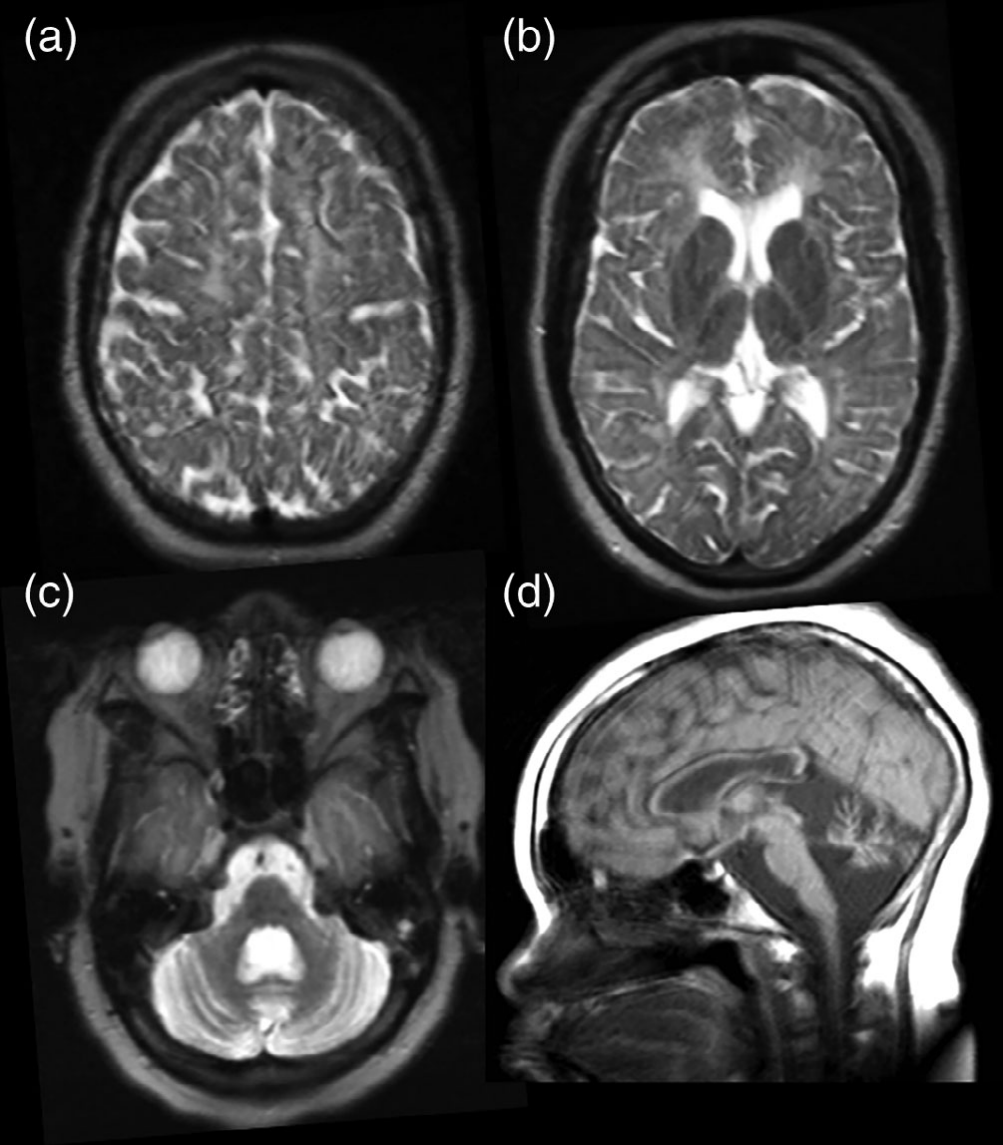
This brain MRI was performed at the age of 17 years. (a–c) are T2‐weighted axial images, demonstrating diffuse hyperintense signal of the white matter, mild supratentorial atrophy, and severe cerebellar atrophy. The ventrolateral thalamus (b) and the medial lemniscus (c) are relatively hypointense, as often seen in 4H leukodystrophy. The sagittal T1‐weighted image (d) demonstrates cerebellar atrophy and a thin corpus callosum
